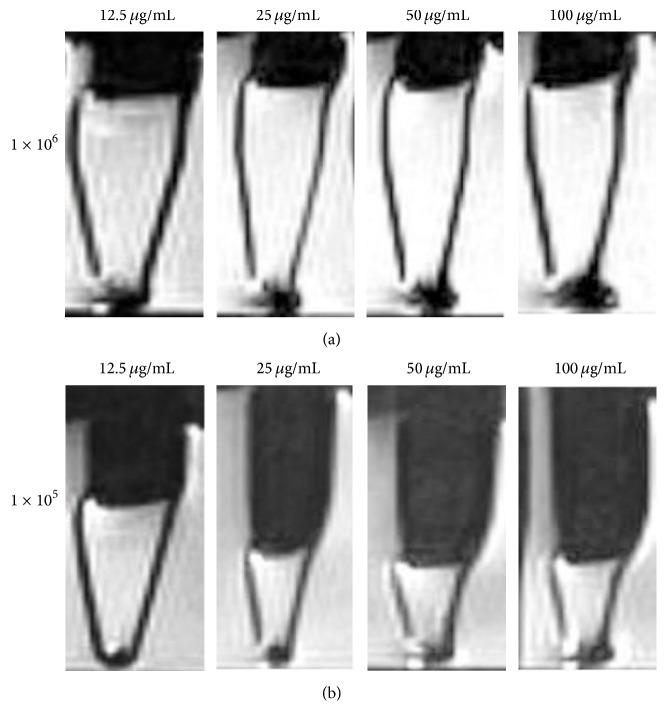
MRI of MIRB-labeled hDPSCs in vitro. (a) MRI of 1 × 10^6^ cells labeled with various concentrations of MIRB. (b) MRI of 1 × 10^5^ cells labeled with various concentrations of MIRB.
